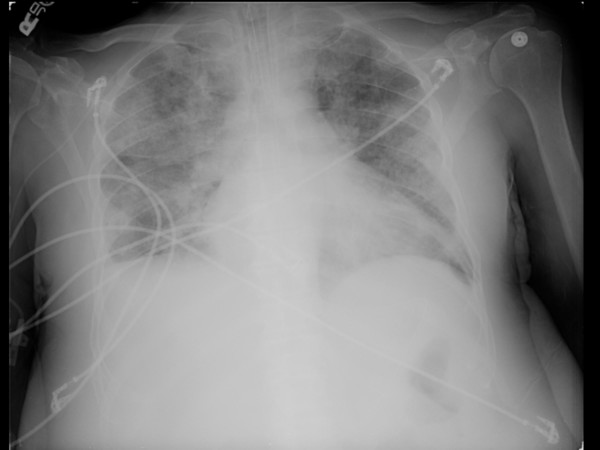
Chest X-ray. Cardiomegaly with diffuse bilateral interstitial infiltrates and a right-sided pleural effusion.
